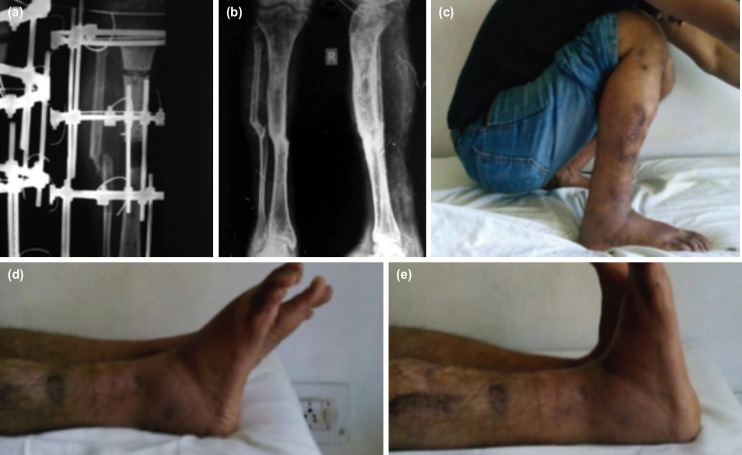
(a) Radiograph during IBT of a case of infected non-union showing regenerate. (b) Final follow-up of the same case after ring removal. (c) Clinical picture at final follow-up showing ROM of knee joint. (d) Clinical picture at final follow-up showing ankle plantar flexion. (e) Clinical picture at final follow-up showing ankle dorsiflexion.
